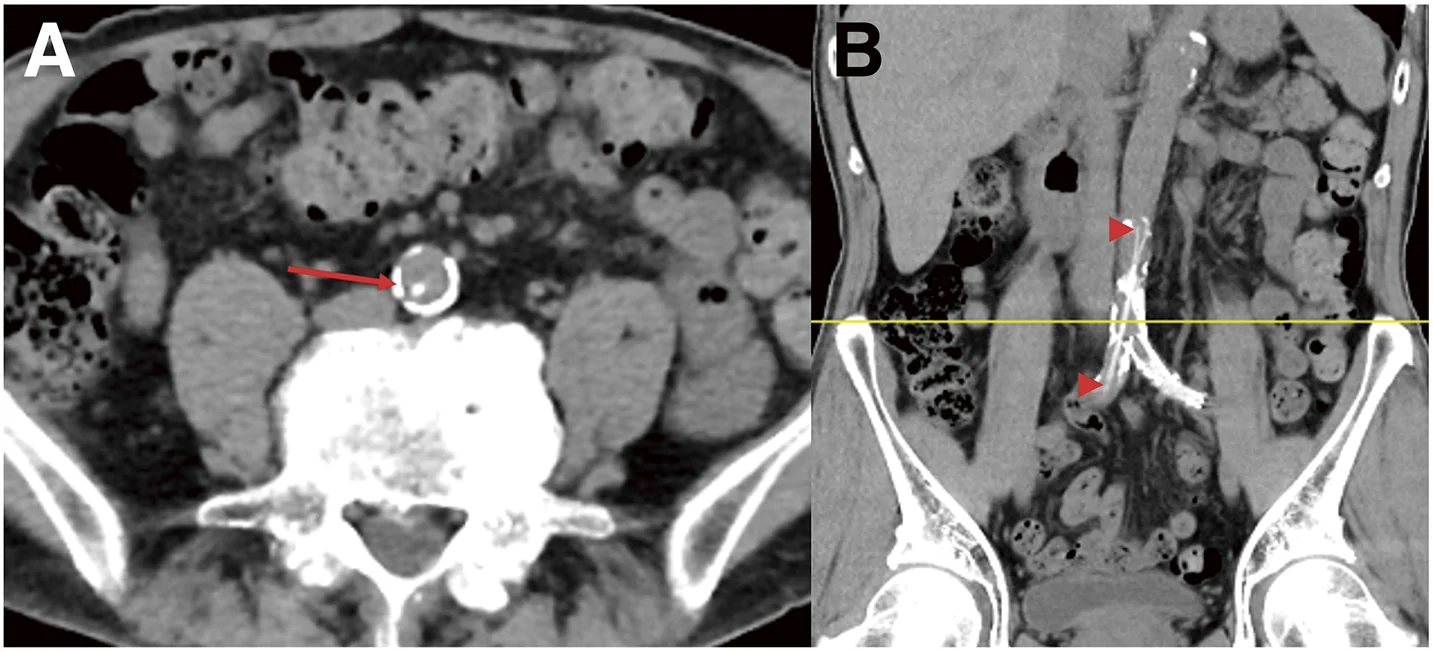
Plain computed tomography of the abdomen. (A) Axial image demonstrating a foreign body consistent with a pigtail catheter straightener (arrow). (B) Coronal image confirming the position of the foreign body between the lower abdominal aorta and the right common iliac artery (arrowheads).
